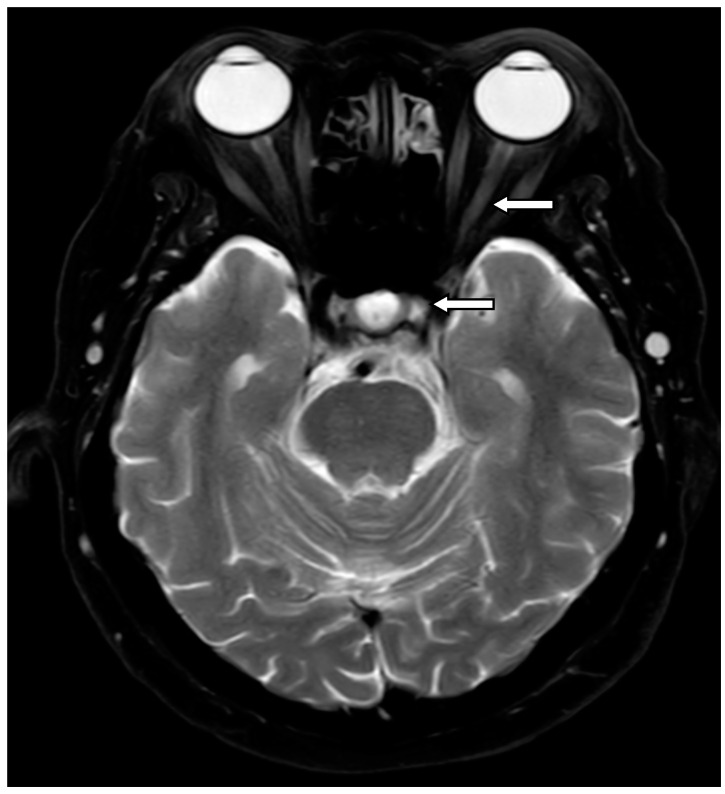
A female patient diagnosed with NMOSD-ON. Her MRI T2W scan showed an enhancing lesion of optic nerve at left intraorbital and optic chiasm (arrows).
